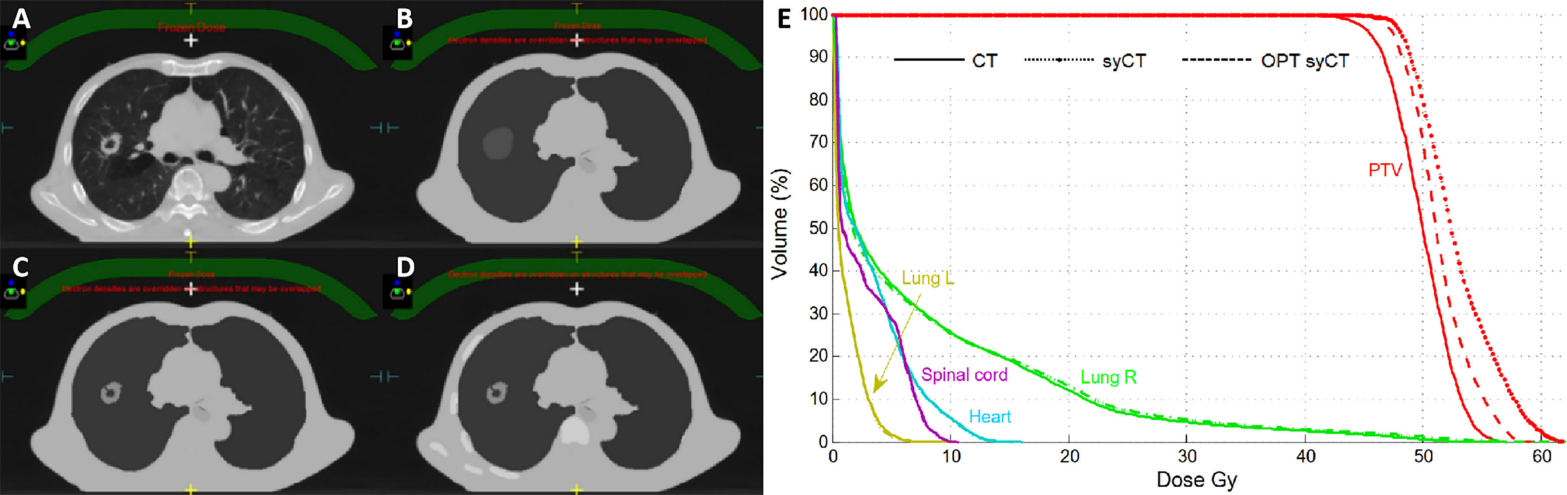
Successive attempts to realign the CT and syCT plan dosimetries for lung case 1. Reference CT representative transversal image (A), corresponding syCT with ED assignments on successive attempts (B–D), and corresponding DVHs (E).
